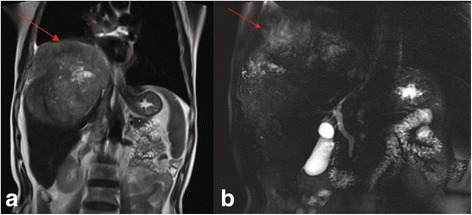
Coronal DCE-MRI of the abdomen shows liver lesions alongside normal bile ducts. a Localized lesions were prominent in the liver capsule, and the right diaphragmatic muscle was raised (arrow). b No dilation of the intrahepatic or extrahepatic bile ducts was observed (arrow)
